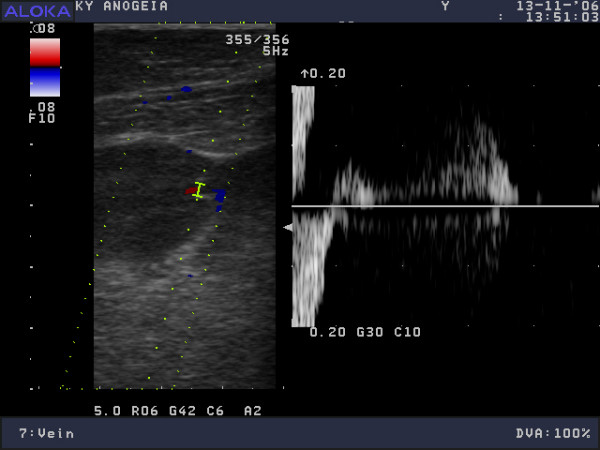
Distal right popliteal vein, colour Doppler ultrasonogram, spectral analysis during the calf muscle squeeze test.
